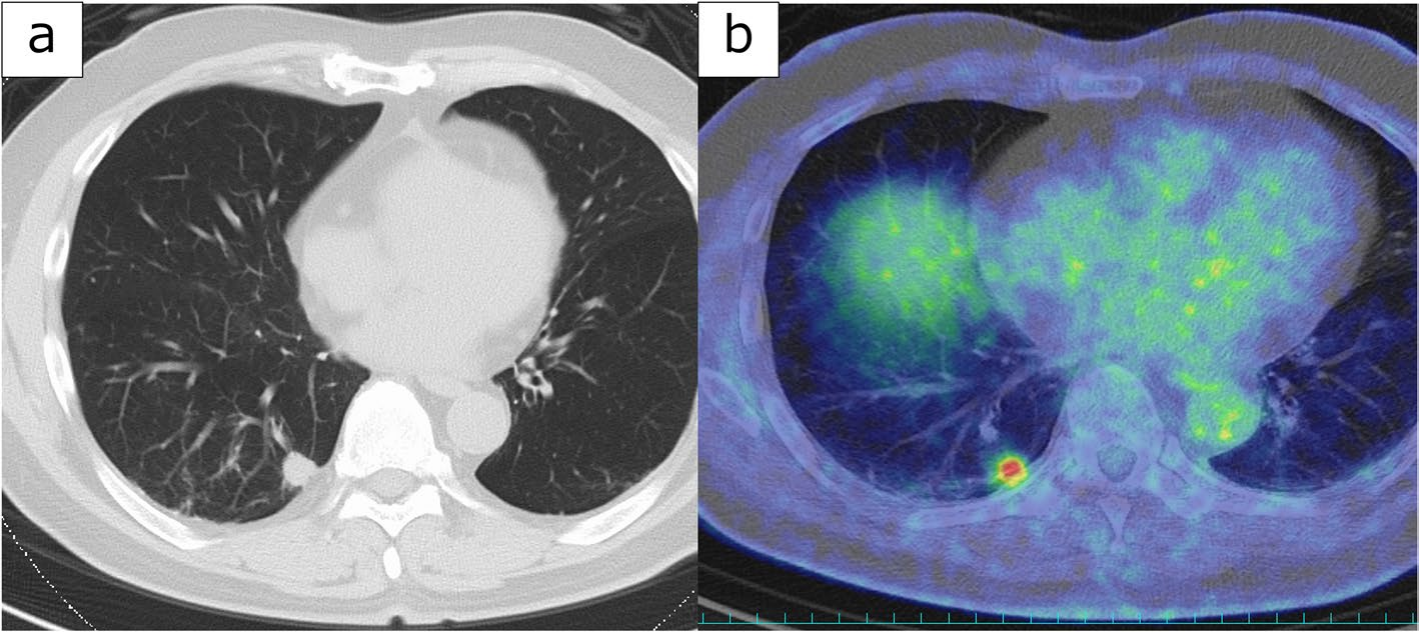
a Chest CT at initial presentation revealed an 18 × 15 mm nodule in the right lower lobe. b PET-CT showed high uptake in the nodule
